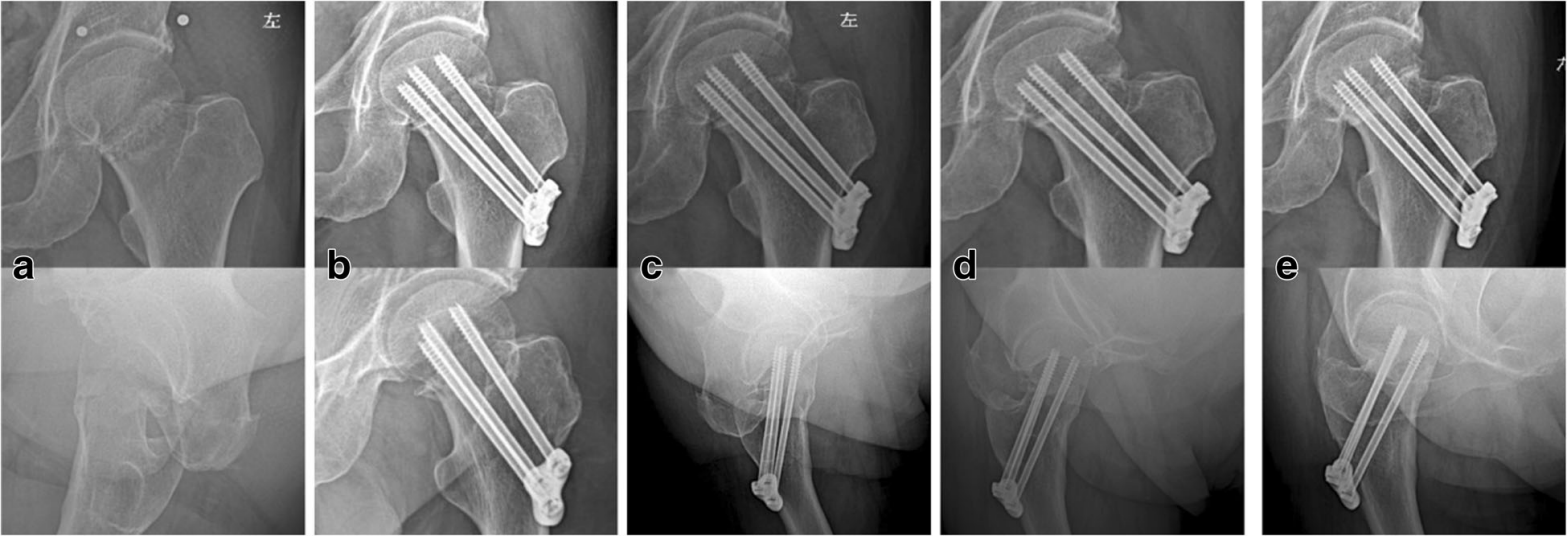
Radiograph showing slight screw back-out. A 67-year-old man fixed with dynamic compression locking system without the irritation of the soft tissue around the plate. a Preoperative X-ray. b Postoperative X-ray review at 1 day after operation. c Postoperative X-ray review at 2 months after operation. d Postoperative X-ray review at 8 months after operation. e Postoperative X-ray review at 20 months after operation
